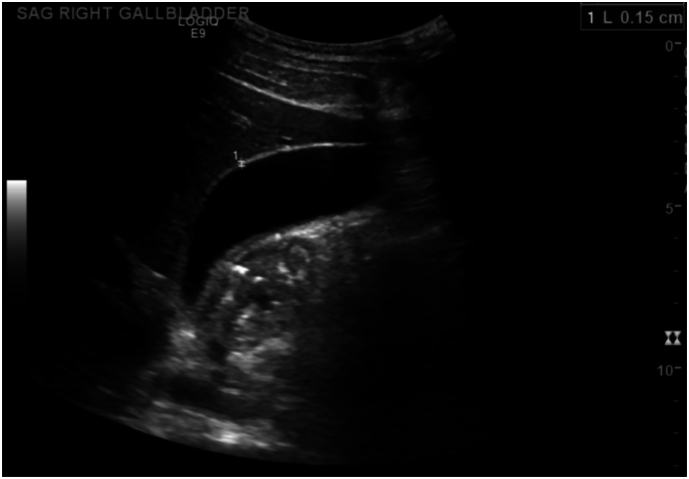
Abdominal ultrasound showing a normal gallbladder wall with absence of gallstones and pericholecystic fluid.
